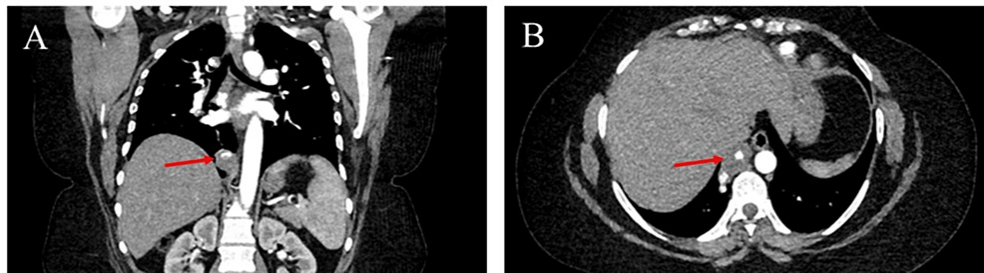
CT angiography of the pulmonary sequestration Computed tomographic angiography demonstrates pulmonary sequestration with an aberrant aneurysmal arterial supply in the (A) sagittal and (B) coronal views.
